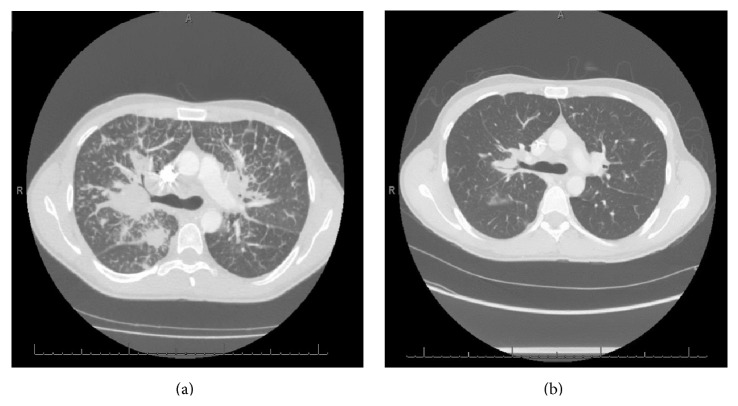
CT chest with large masses bilaterally at level of bifurcation of trachea (a) and after 4 cycles of VAC/IE chemotherapy (b).
